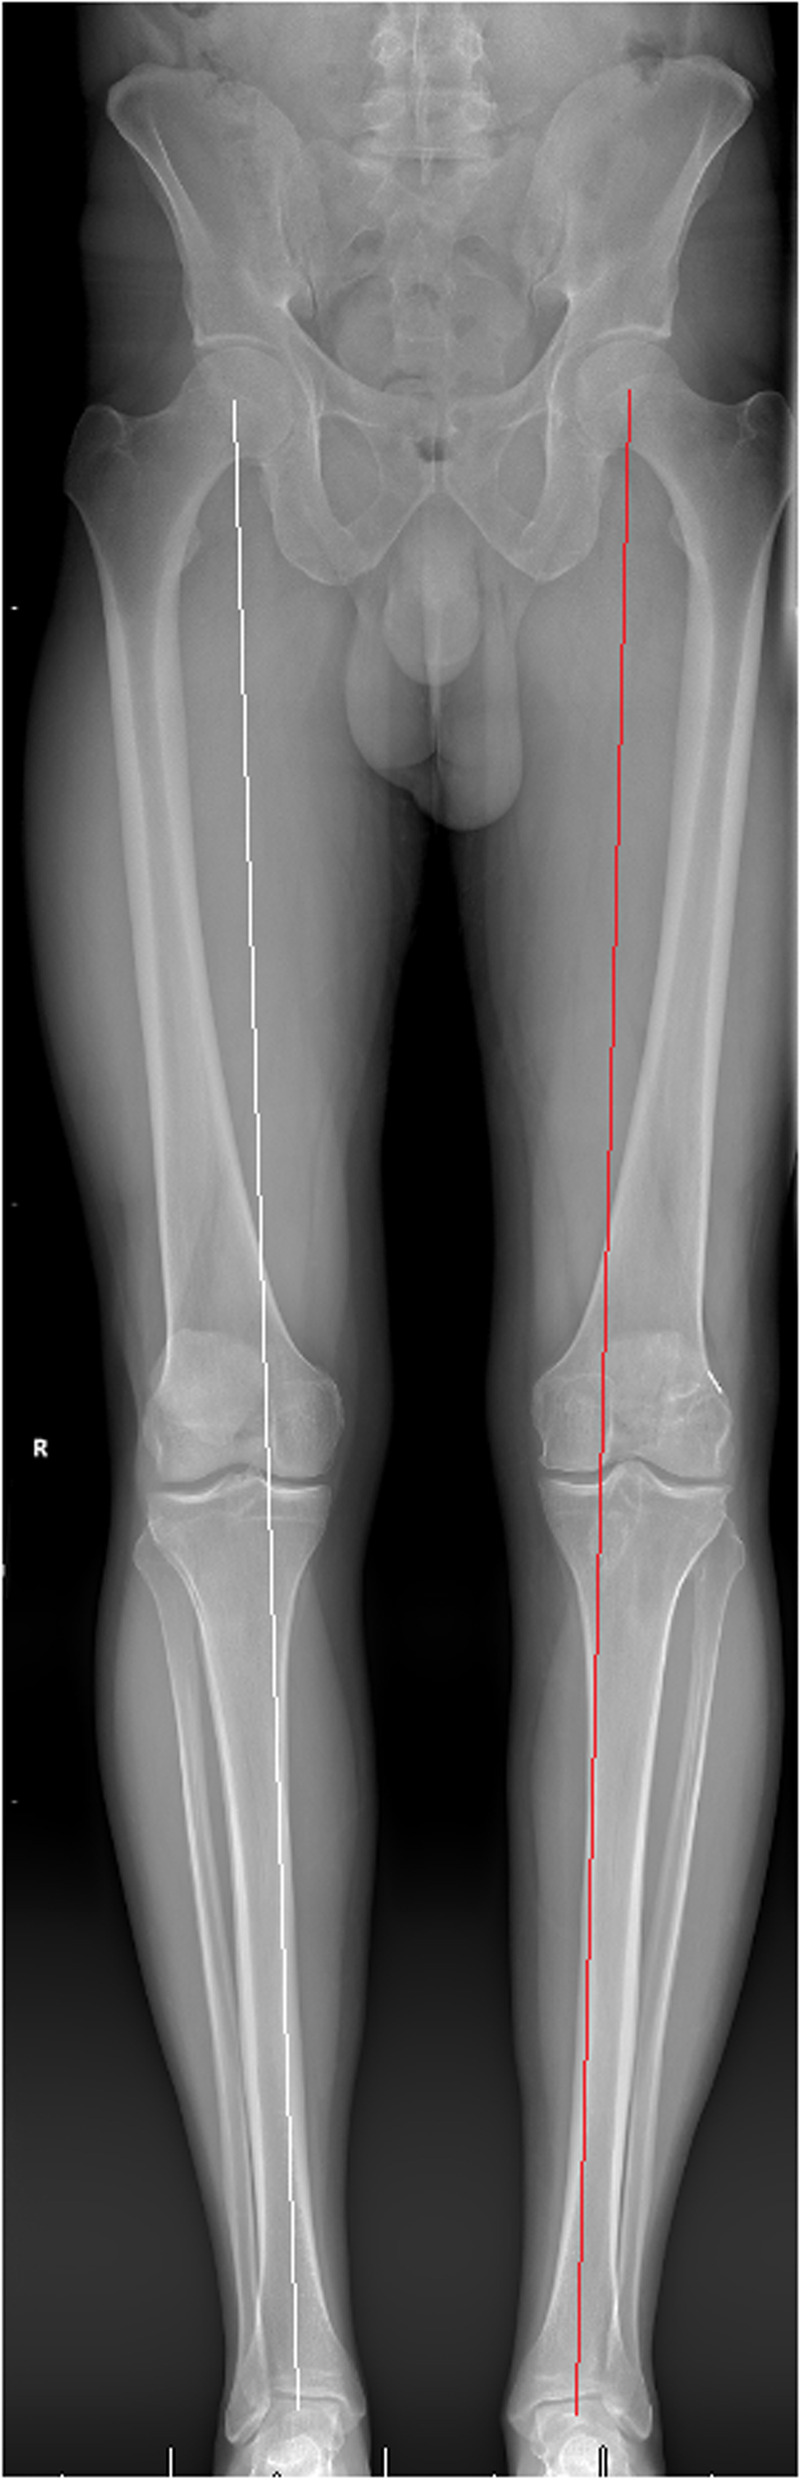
A pre-operative standing mechanical axis radiograph demonstrating slight native varus alignment
